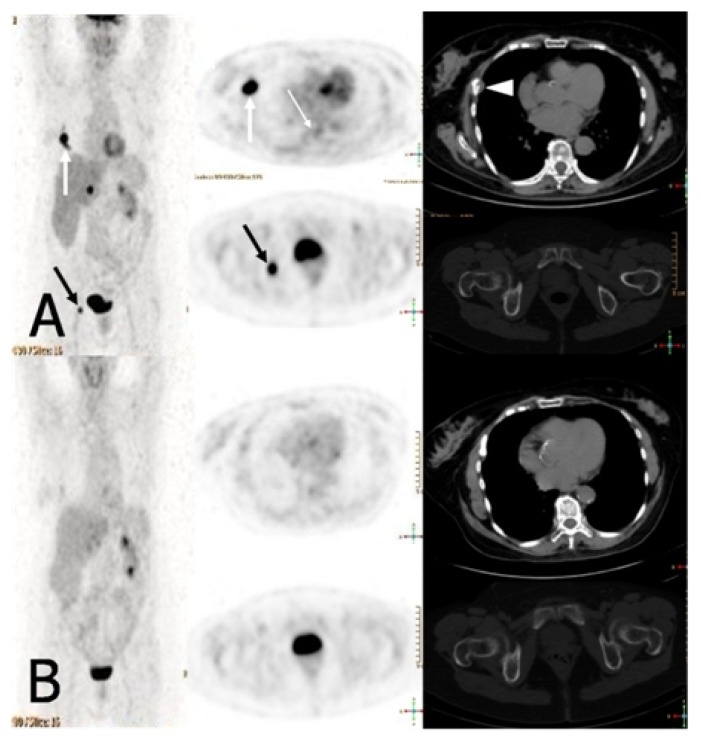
A. PET showing three focal lesions in the fifth right rib (*thick white arrows*), D10 vertebral body (*thin white arrow)*, and right ischium (*black arrow)*. Only the lesion of the fifth right rib was evident at CT images (*arrowhead)*. B. PET showing complete disappearance of the lesions after lenalidomide therapy.
